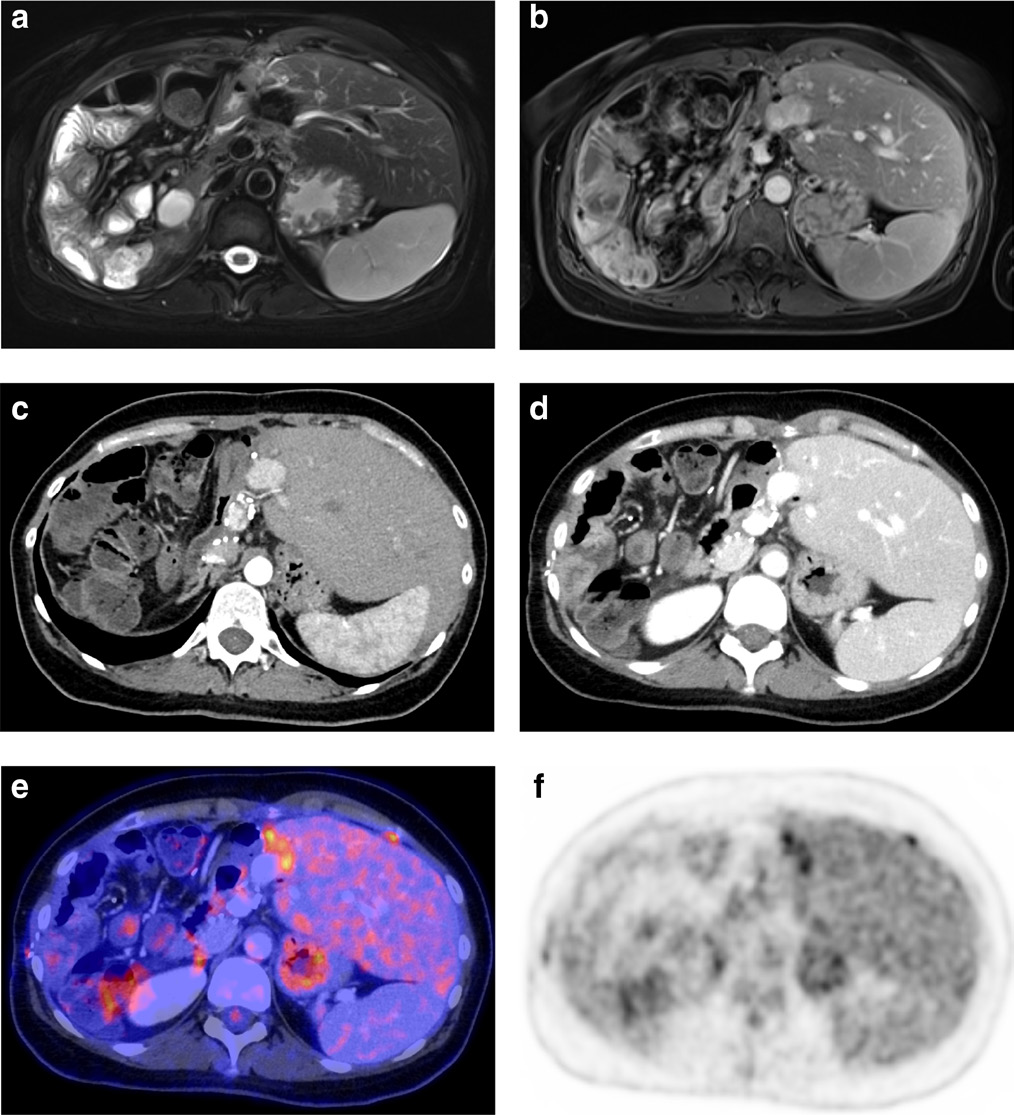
Example of a 36-year-old female patient with recurrent CCA in the left liver lobe after right hemihepatectomy and systemic chemotherapy (gemcitabine/cisplatin), not detected on CT and MRI. Recurrence was suspected clinically by elevated tumor markers (CA 19.9). Before PET/CT, clinicians intended further imaging and biopsy to prove suspected recurrence, and revised their “non-treatment” strategy into a “palliative treatment” goal (with change of chemotherapy regimen) after PET/CT (*major change*).
